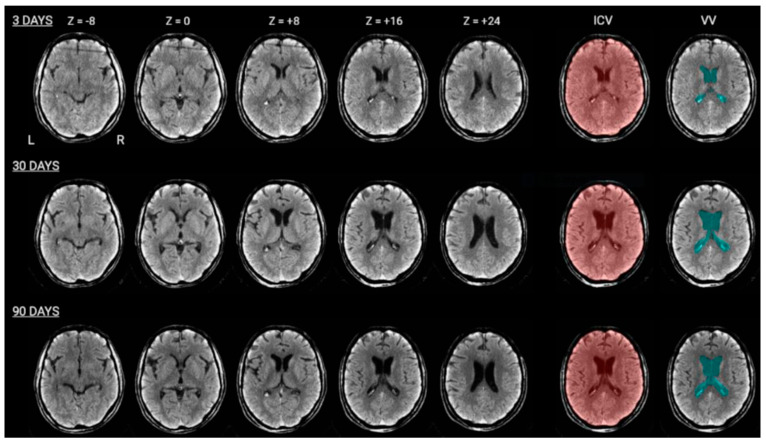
CT scans of the same patient obtained at 3 days (first line), 30 days (second line) and 90 days (third line) after injury. The Z values refer to the coordinate of the horizontal sections in the MNI brain space. The ICV column shows the automatically colored selection of the intracranial volume. The VV columns show the ventricle volume collected automatically and controlled by visual inspection.
